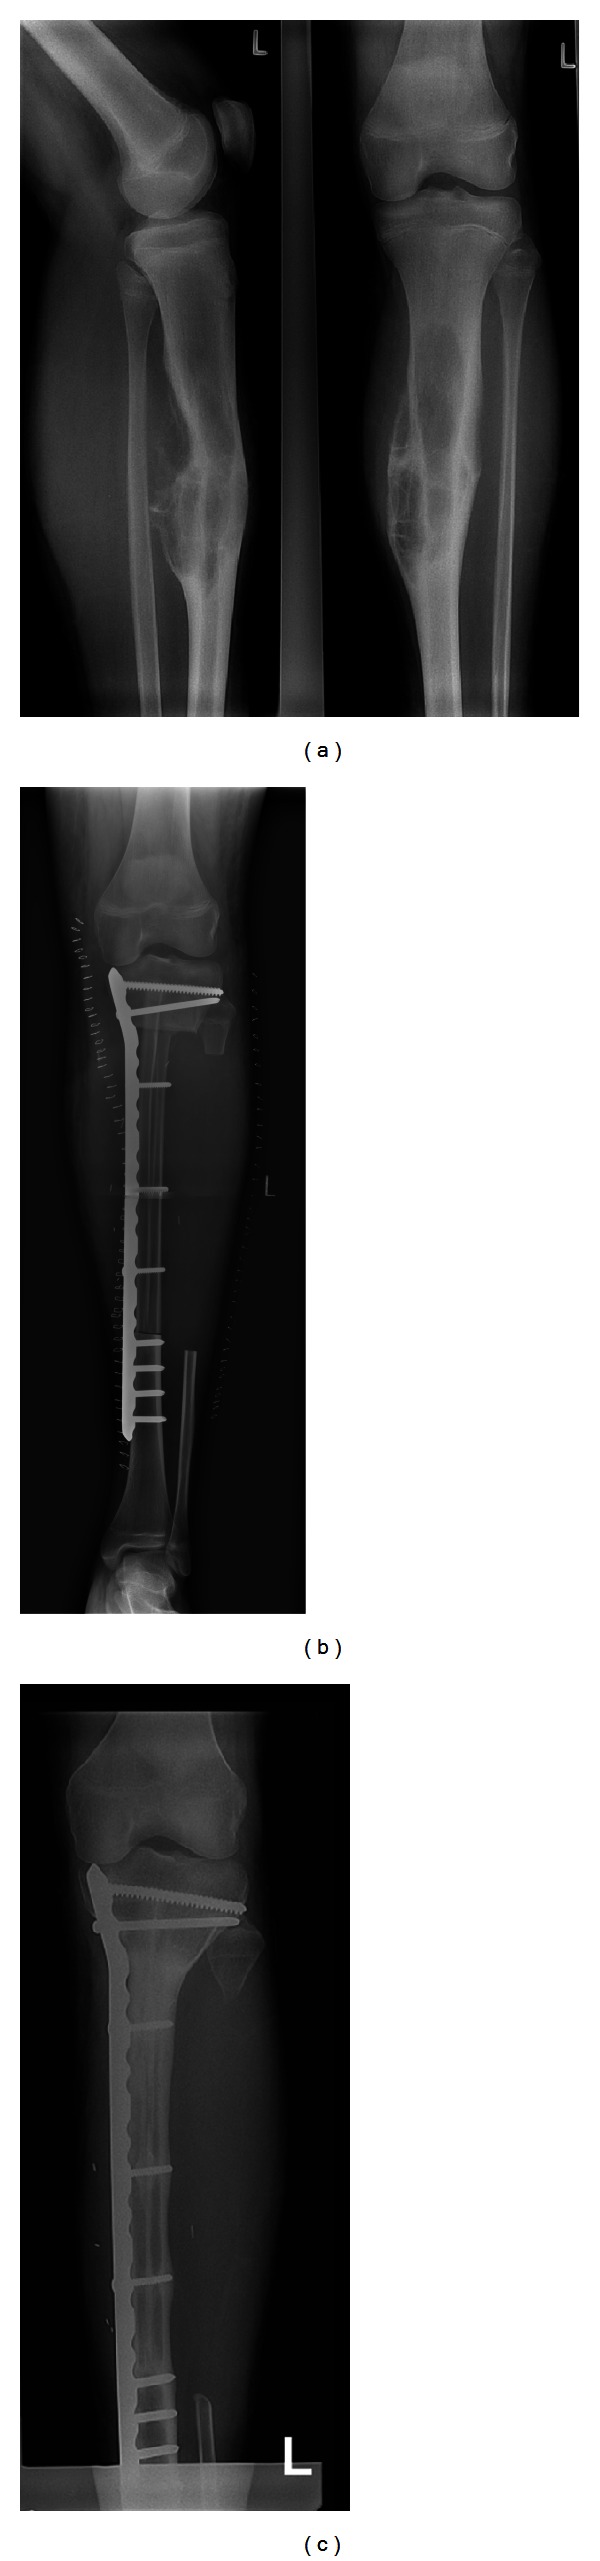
(Patient no. 9) (a) 14-year-old boy with Ewing's sarcoma of the left tibia proximal diaphysis. (b) After completion of neoadjuvant chemotherapy wide resection and reconstruction of the defect (24.5 cm) by a vascularized transposed ipsilateral and contralateral free fibula and medial plate fixation were realised. (c) Radiograph showing osseous integration and hypertrophy of fibular grafts 15 months after operation.
